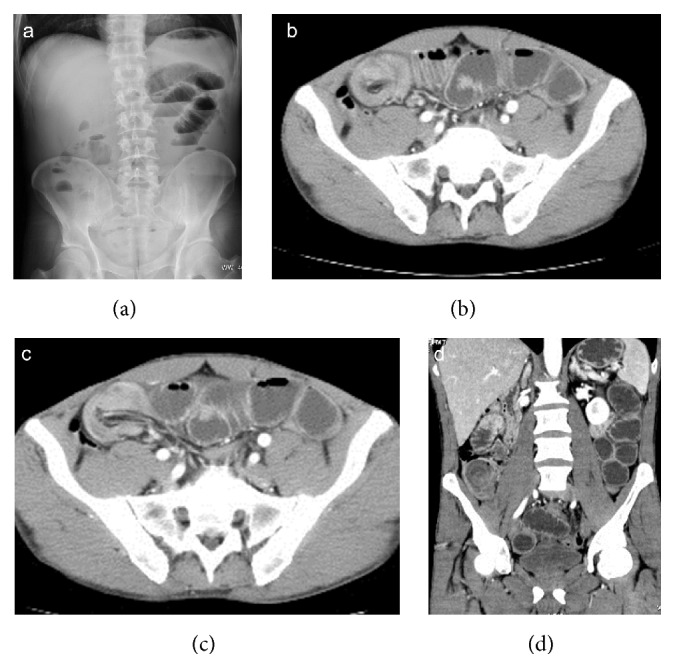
Enterocolonic (ileocaecocolonic) intussusception caused by intestinal lymphoma, with proximal bowel obstruction. Plain abdominal radiography (a) shows gas-fluid levels within distended small bowel loops. Intussusception is well depicted on axial CT scans (b, c) and coronal reformatting (d).
